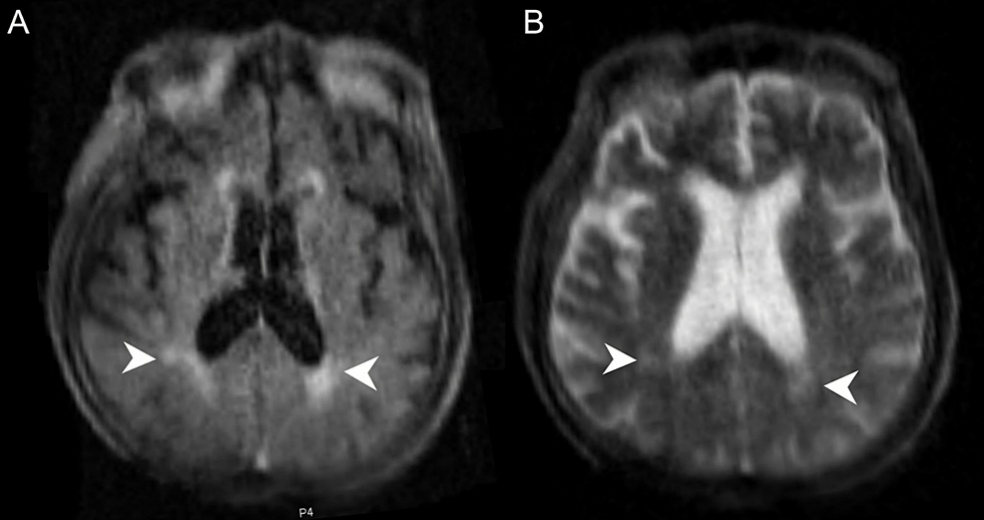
FLAIR (A) and T2-weighted (B) images from a portable brain scan using a 0.064T magnet highlights small white matter changes along the periventricular margin (arrowheads). FLAIR, fluid-attenuated inversion recovery.
